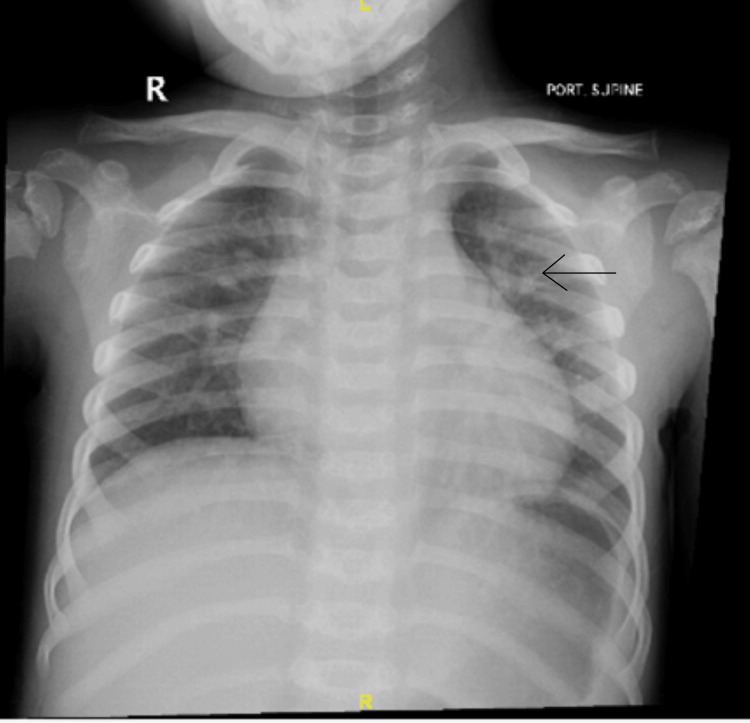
Chest X-ray Left retrocardiac airspace opacity which could represent consolidation/atelectasis, no pneumothorax or pleural effusion with normal cardiac silhouette.
